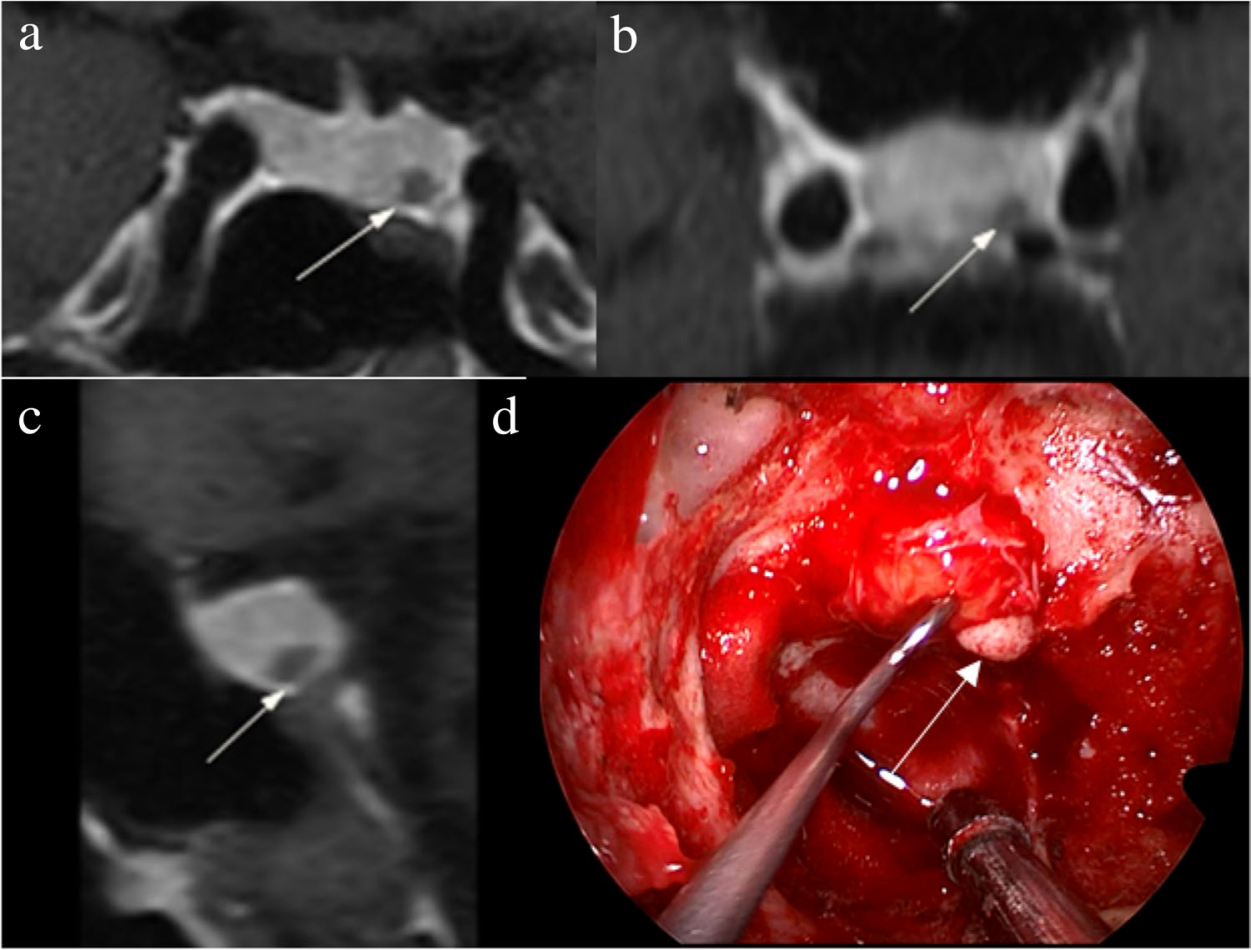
Images in a 48-year-old woman with Cushing’s disease. Preoperative high-resolution contrast-enhanced MRI using three-dimensional fast spin echo sequence shows a 4-mm pituitary microadenoma (arrow) with delayed enhancement is well delineated on the left side of the pituitary gland on (a) coronal, (b) reconstructed axial, and (c) reconstructed sagittal contrast-enhanced T1-weighted images. d Intraoperative endoscopic photograph during transsphenoidal surgery after exposure of the sellar floor shows a round pituitary microadenoma (arrow)
